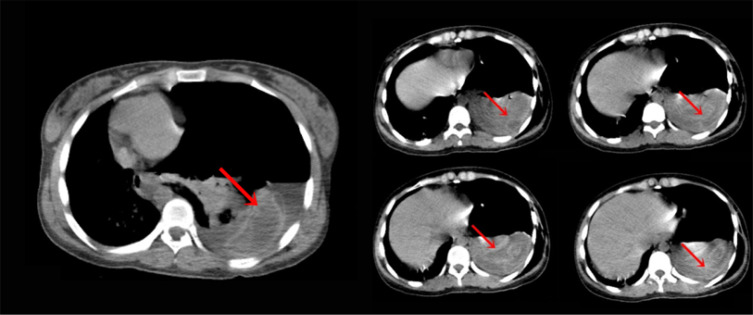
Axial computed tomography (CT) scan in the mediastinal window of patient, showing crumpled laminated membrane (red arrows).
